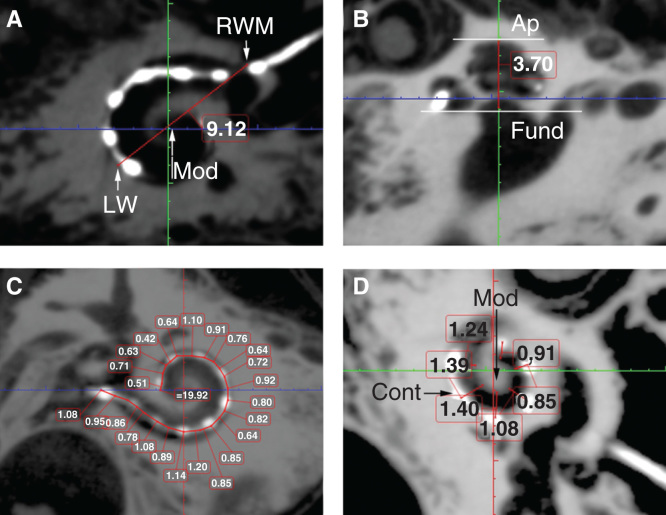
Critical cochlear assessments on cone-beam computed tomography (CBCT) images. ‘A’ value (A), cochlear height (B), insertion depth for the first 360° insertion angle (C), and distance between the electrode contacts and the modiolus (D) are demonstrated (in mm). Ap, apex; Cont, contours of cochlear implant; Fund, fundus; LW, lateral wall; Mod, modiolus; RWM, round window membrane.
